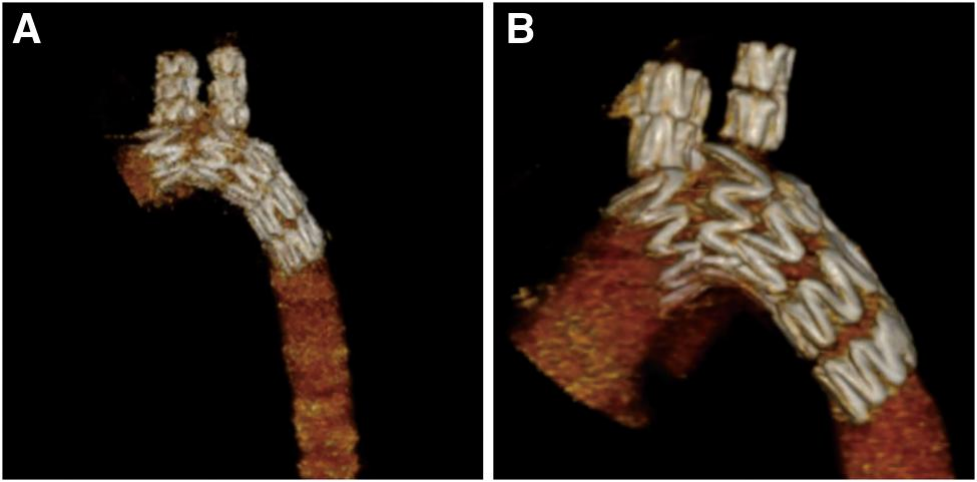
Three-dimensional computed tomography angiography images revealed no rupture, fracture, twist or migration in the main stent and in the sidearm branches. (A) At 90 days follow-up; (B) at 180 days follow-up.
